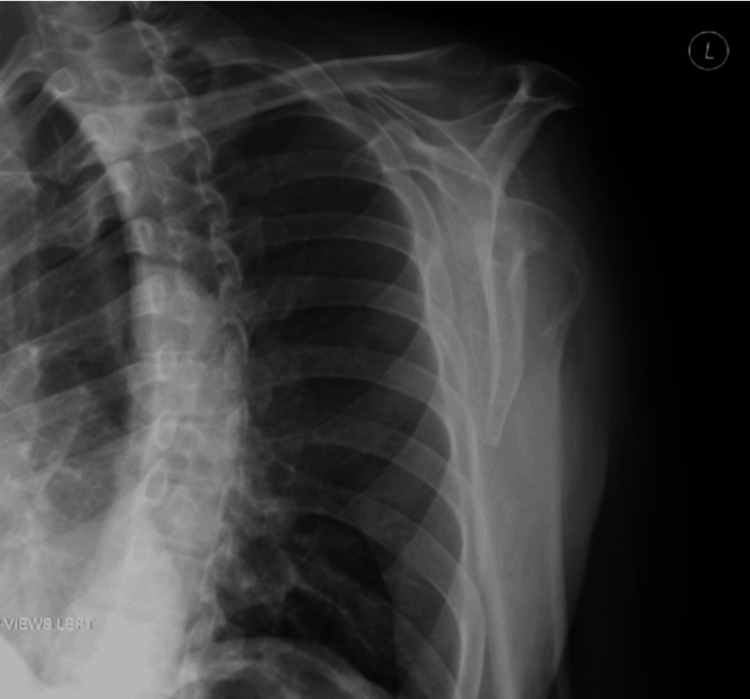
X-ray of LUE showing triceps tendon insertional enthesopathy and inferior subluxation of humeral head after significant proximal muscle atrophy. LUE: Left upper extremity
